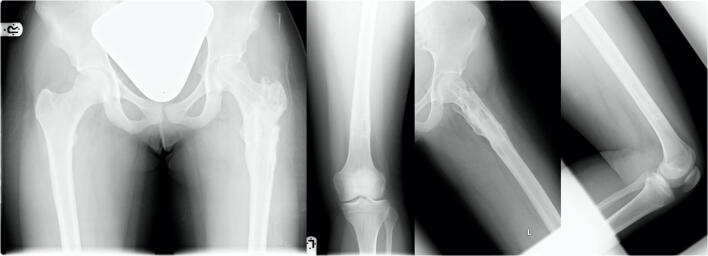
Anterior-posterior view of the pelvis; anterior-posterior and axial X-ray of the left femur healed fracture at the latest follow-up (4 years after injury) after implant removal
